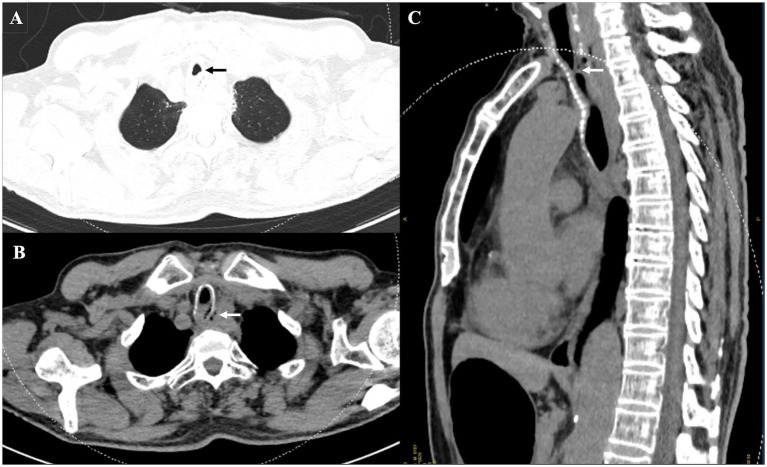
Serial chest computed tomography (CT) imaging at the external hospital. (A) Axial CT scan (lung window): moderate cervical tracheal stenosis (black arrow). (B,C) Axial and sagittal CT scans (mediastinal window): focal wall thickening of the cervical and upper thoracic esophagus (~1.5 cm), with obliteration of periesophageal fat planes, indistinct tracheal borders, and moderate cervical tracheal stenosis (white arrow). The lesion invades the tracheal lumen with a focal tracheoesophageal fistula, demonstrating the air-containing cavity (white arrowhead).
